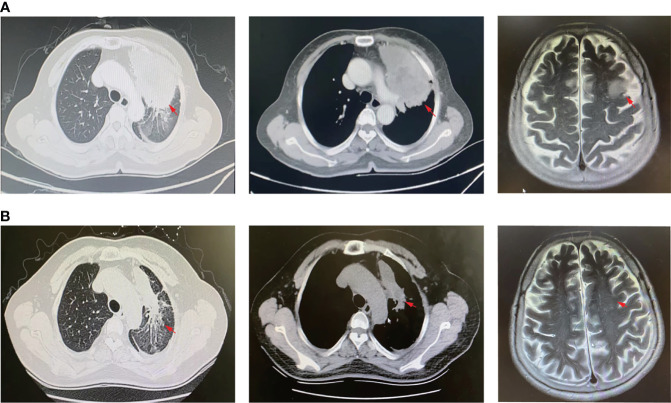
CT scan and MRI images. (A) CT scan of lung lesions (left and middle panel) and MRI profile (right panel) before Savolitinib treatment. (B) CT scan of lung lesions (left and middle panel) and MRI profile (right panel) 2-months post Savolitinib treatment. The red arrow indicates the location of the lesion.
